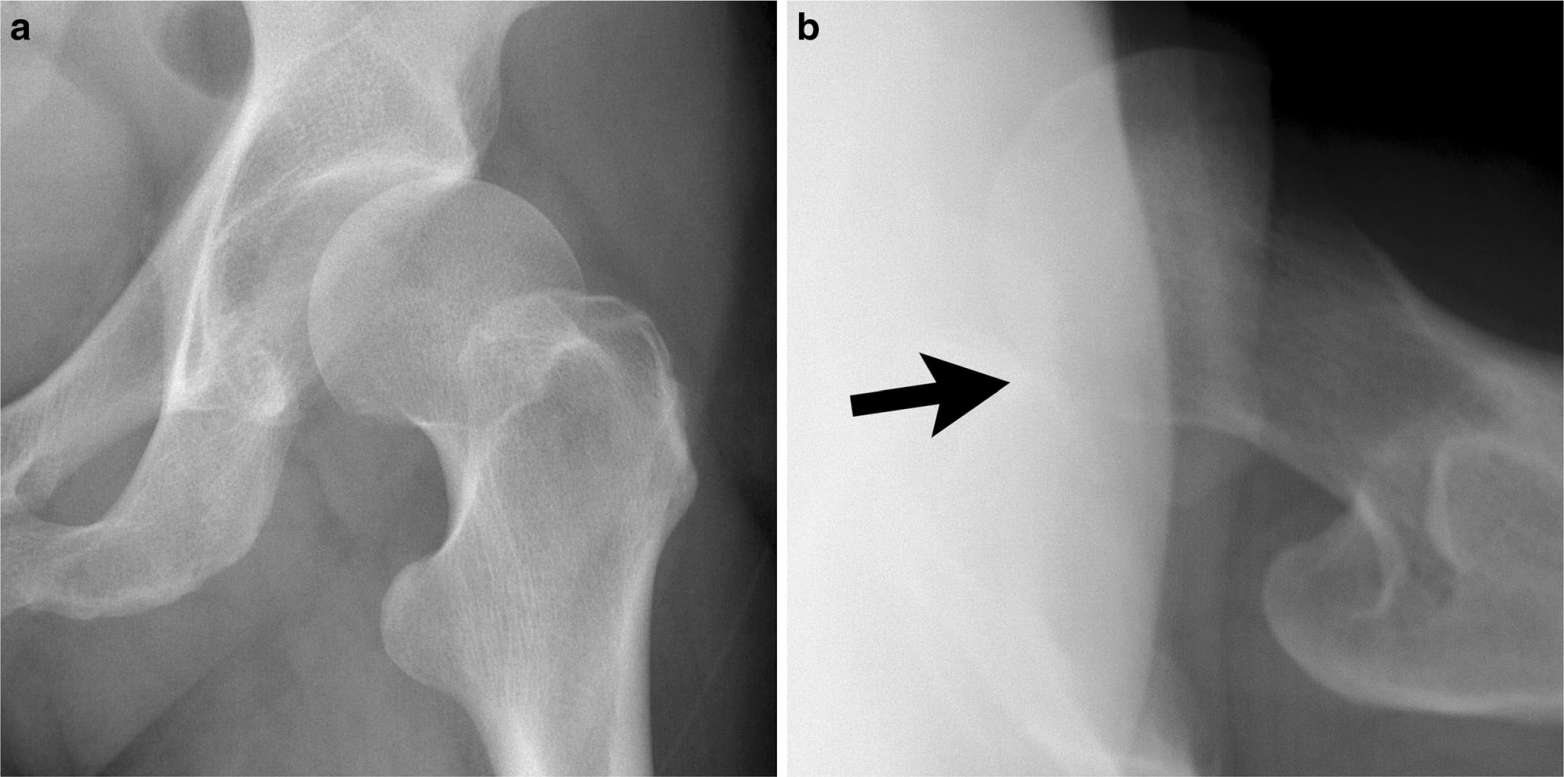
A 50-year-old man had an atraumatic anterior hip dislocation following arthroscopy for FAI. He subsequently underwent total hip arthroplasty. a AP radiograph shows the femoral head perched on the rim of the acetabulum. b Cross table lateral radiograph confirms that the dislocation is anterior. The femoral head is perched on an anterior acetabular rim (*arrow*)
